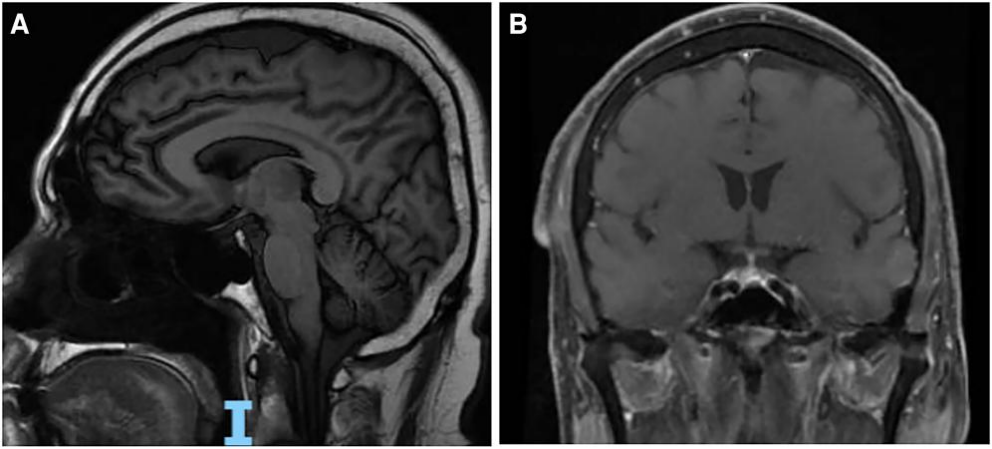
Brain MRI without any evidence of metastasis or hypophysitis.
